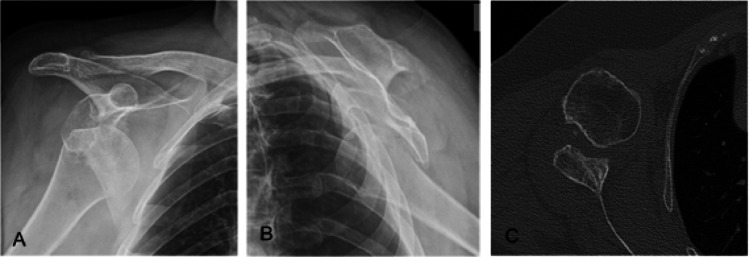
A and B AP and transscapular X-rays view. C CT scan showing chronic shoulder dislocation with anteroinferior bone defect
